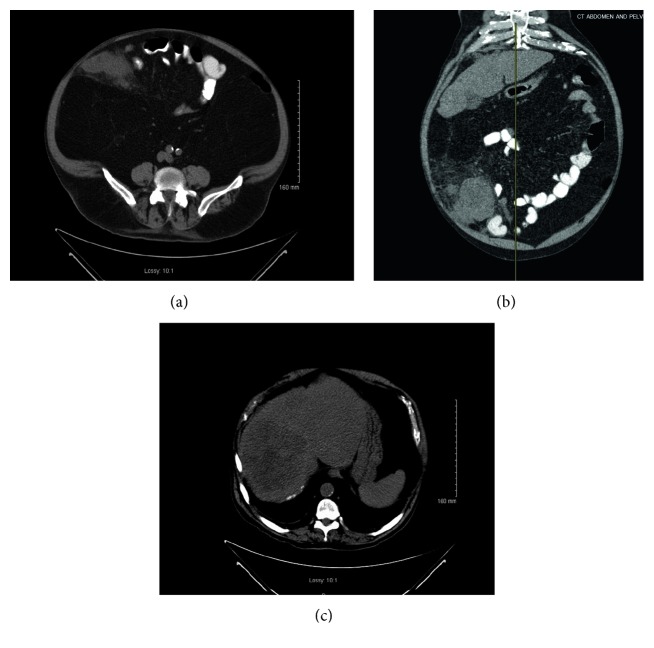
CT scan of the abdomen and pelvis. (a) Axial view of the large tumor in the cecum. (b) Coronal view of the tumor with surrounding inflammatory changes. (c) Large lesion in the right lobe of the liver also seen on CT scan suggestive of metastatic disease.
